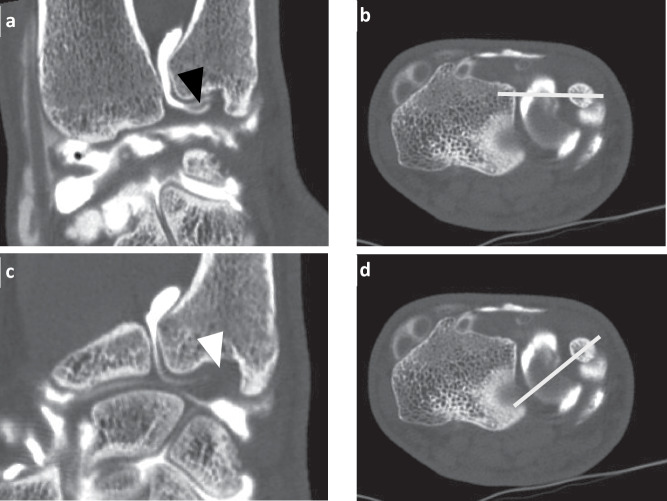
Arthro-CT of a 31-year-old woman with ulnar-sided wrist pain and no radioulnar instability; no lesions were described in the initial report. The review shows a partial foveal tear with preservation of the styloid bundle. a, b Dorsal foveal fissure visible on a dorsal radial plane (black arrow), corresponding to the white line on axial view. c, d Intact appearance of the volar portion of the foveal attachment (white arrow) on a volar radial plane
